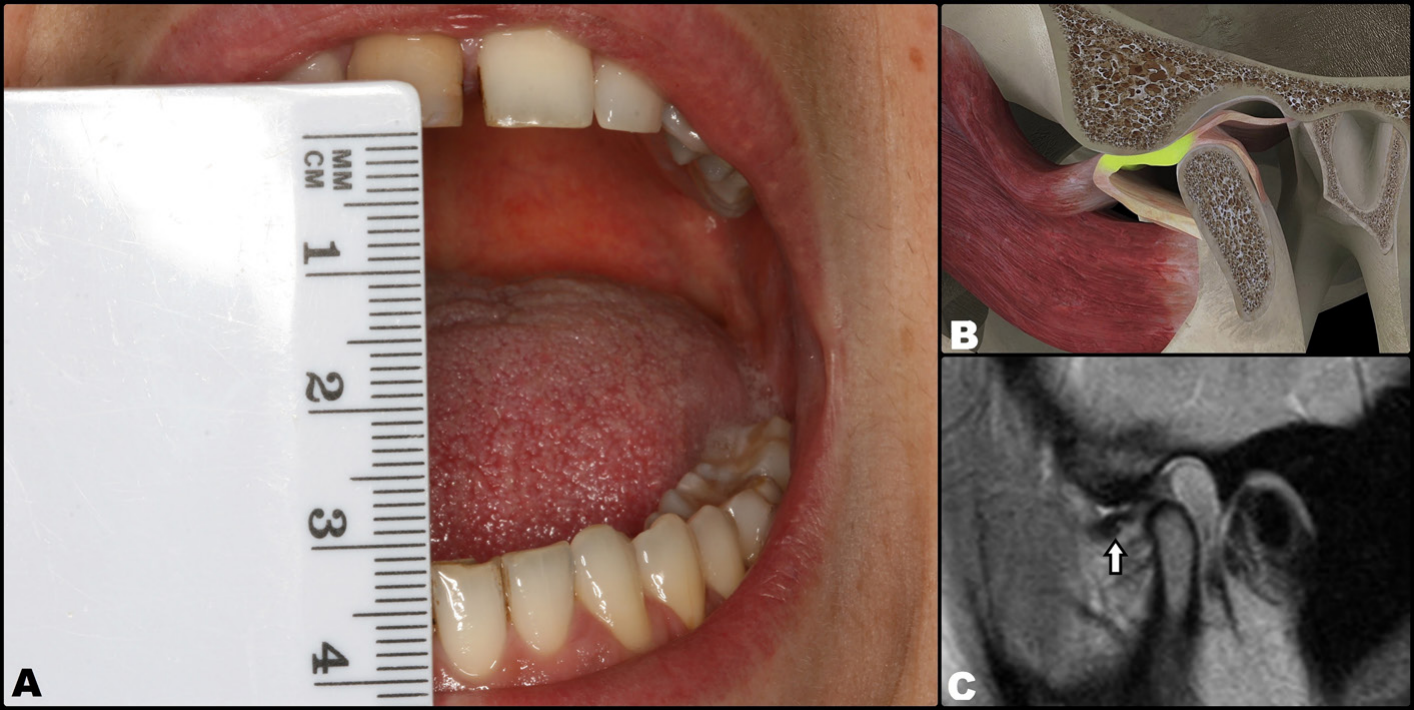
Initial situation. Disc displacement without reduction and with limited opening of the mouth of the patient. A: Opening limited to 30 mm. B: Three-dimensional representation at intra-articular level. C: Pre-treatment magnetic resonance imaging view (arrow: disc displacement).
